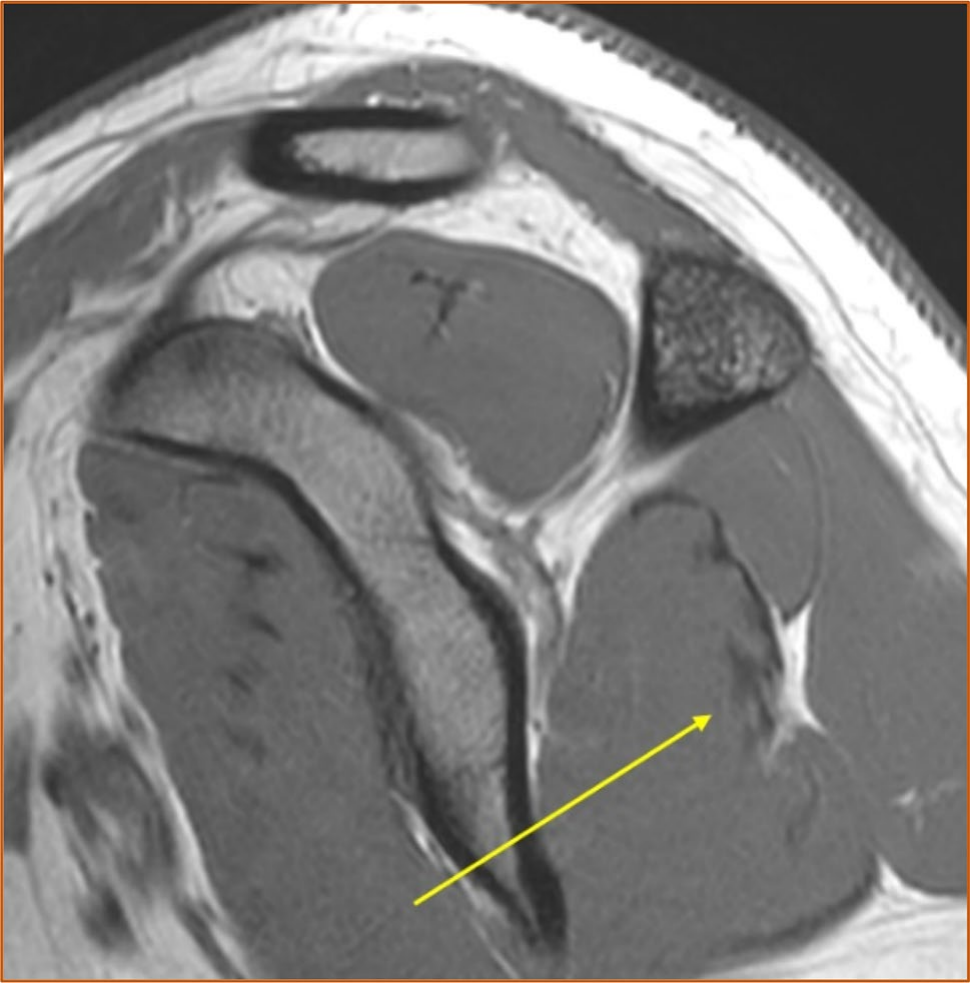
Example of intramuscular tendons that were judged as partly superficial, T1-weighted image. Sagittal section
